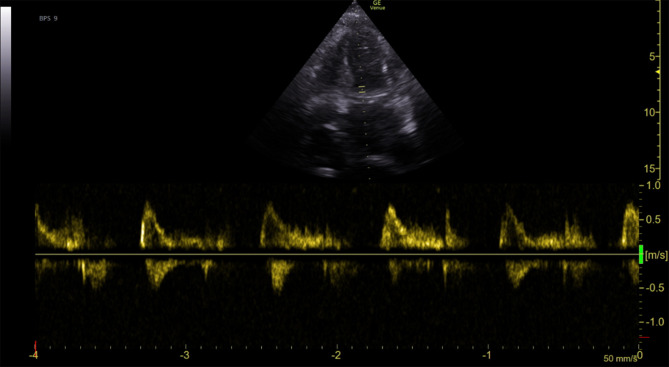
Transthoracic echocardiography showing a hypertrophic left ventricle with hyperdynamic systolic function and restrictive filling pattern
